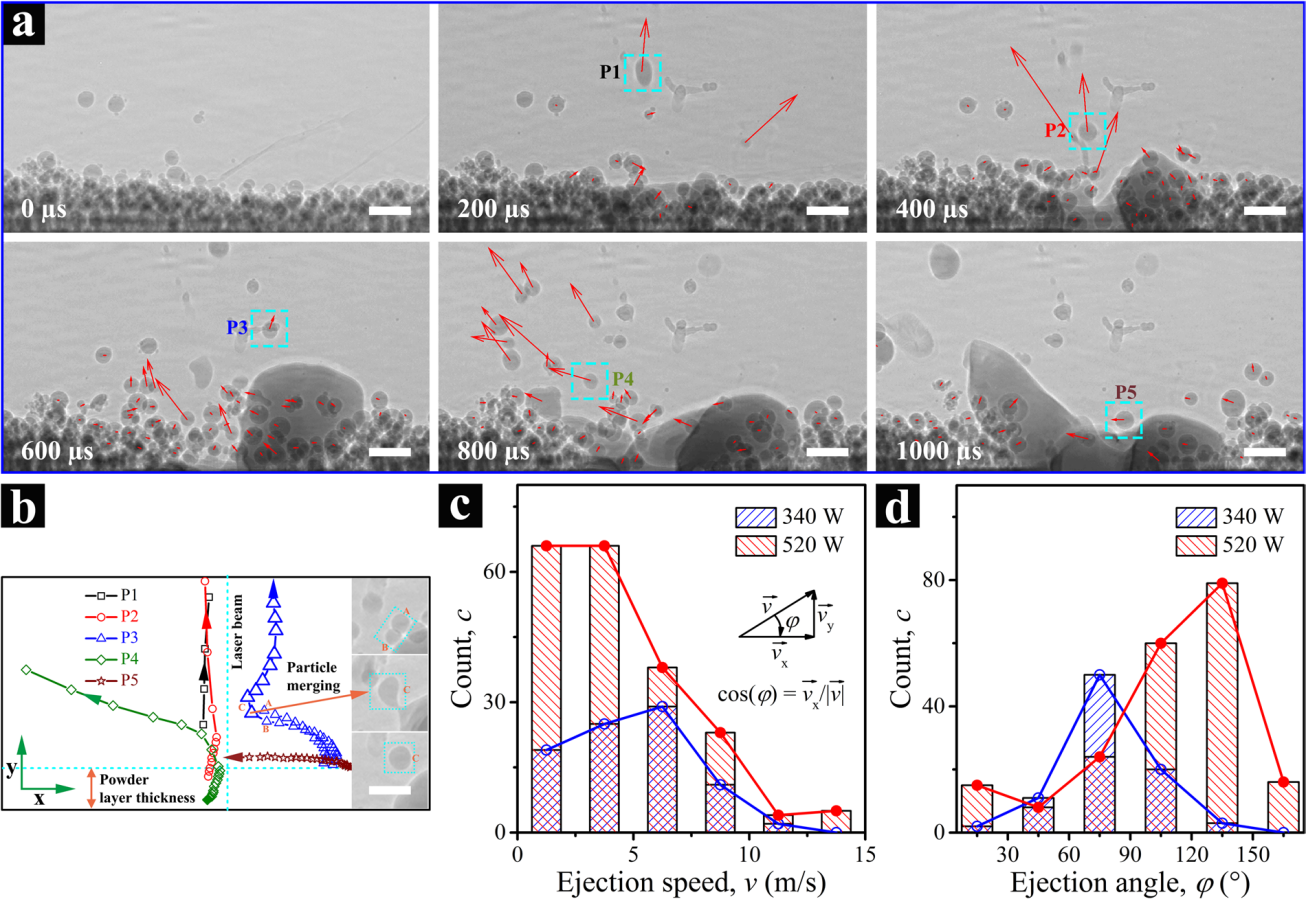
Tracking of powder motion during the laser powder bed fusion process of Ti-6Al-4V. (a) Dynamic X-ray images collected at different times, with red arrows indicating the velocity of each individual powder particle. In this case, the laser power and beam size are 520 W and ~220 µm (1/e^2^), respectively. The particle size is in the range of 5–45 µm, and the powder layer thickness is ~100 µm. The full tracking procedure for two laser power conditions are shown in Supplementary Videos 5 and 6. (b) Moving trajectories of five different particles P1–P5, which are marked out using cyan dashed squares in (a). The dimensions of (b) and each image in (a) are identical. The vertical and horizontal cyan dashed lines specify the location of the laser beam and the top surface of the powder bed, respectively. The insets of (b) show a particle merging process. All the scale bars in (a) and (b) are 100 µm. (c,d) Statistics of the powder moving velocity. Distributions of (c) ejection speed and (d) ejection angle of particles outside the powder bed under the laser power of 340 W and 520 W are shown in blue and red colors, respectively.
